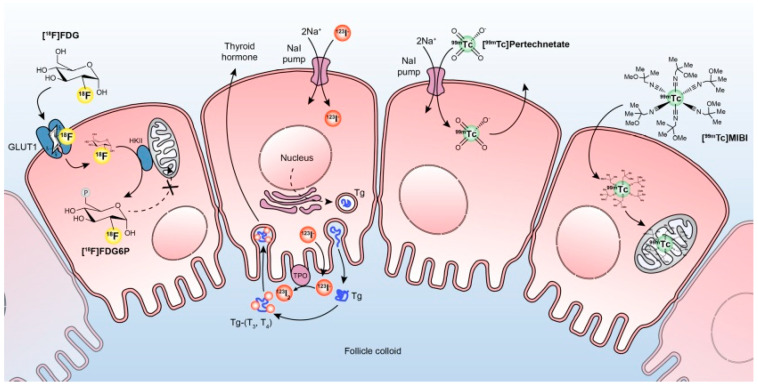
The different metabolic pathways in radioisotope imaging of thyroid nodules. ^18^F FDG is transported into the follicular cell by the transmembrane glucose transporter GLUT1. The intracellular ^18^F FDG is then phosphorylated by HKII to ^18^F FDG6P, which traps the compound and prevents its efflux. In malignant cells, both the expression of GLUT1 transporters and the activity of hexokinase may be increased. Thyroid follicular cells trap ^123^I using the sodium-iodine symporter, which concentrates iodine intracellular and incorporates the ^123^I into thyroglobulin (Tg). The ^123^I is oxidized by thyroid peroxides’, at the follicular cell colloid interface, to neutral iodine, which binds to tyrosine residues on Tg to form thyroid hormones (T3 and T4) stored in the colloid follicular lumen. A similar mechanism is encountered for other iodine radiotracers like ^124^I and ^131^I. ^99m^TcO_4_^−^ is trapped by the thyroid follicular cells in an identical manner as ^123^I, but is neither organified nor incorporated into thyroid hormones and then is not retained in the thyroid. ^99m^Tc MIBI is a lipophilic monovalent cationic agent that crosses the cell membrane and concentrates in the mitochondria due to its positive electric potential. Adapted from Rager O et al. Gland Surg 2019 [6]. Copyright ID: 1187094-1, Nancy International Ltd. Subsidiary AME Publishing Company.
